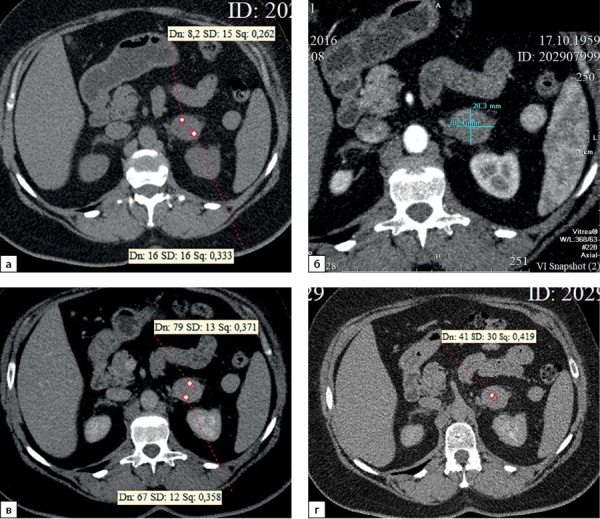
Рисунок 3. Компьютерные томограммы пациентки И., 67 лет с малыми размерами адренокортикальной карциномы (28х35 мм), распространяющейся из латеральной ножки левого надпочечника вдоль передней поверхности почки: а — изображение в аксиальной плоскости, нативная фаза сканирования — показатели плотности до 16 HU; б — артериальная фаза постконтрастного сканирования; в — венозная фаза; г — фаза отсроченного сканирования, коэффициент абсолютного вымывания контрастного препарата составил 42%, относительного — 31,8%.Figure 3. Computed tomography of patient I., 67 years old, with small size adrenocortical carcinoma (28x35 mm), spreading from the lateral pedicle of the left adrenal gland along the anterior surface of the kidney: a — image in the axial plane, native scanning phase — density values up to 16 HU; b — arterial phase of post-contrast scanning; c — venous phase; d — phase of delayed scanning, the coefficient of absolute washout of the contrast agent was 42%, relative — 31.8%.
